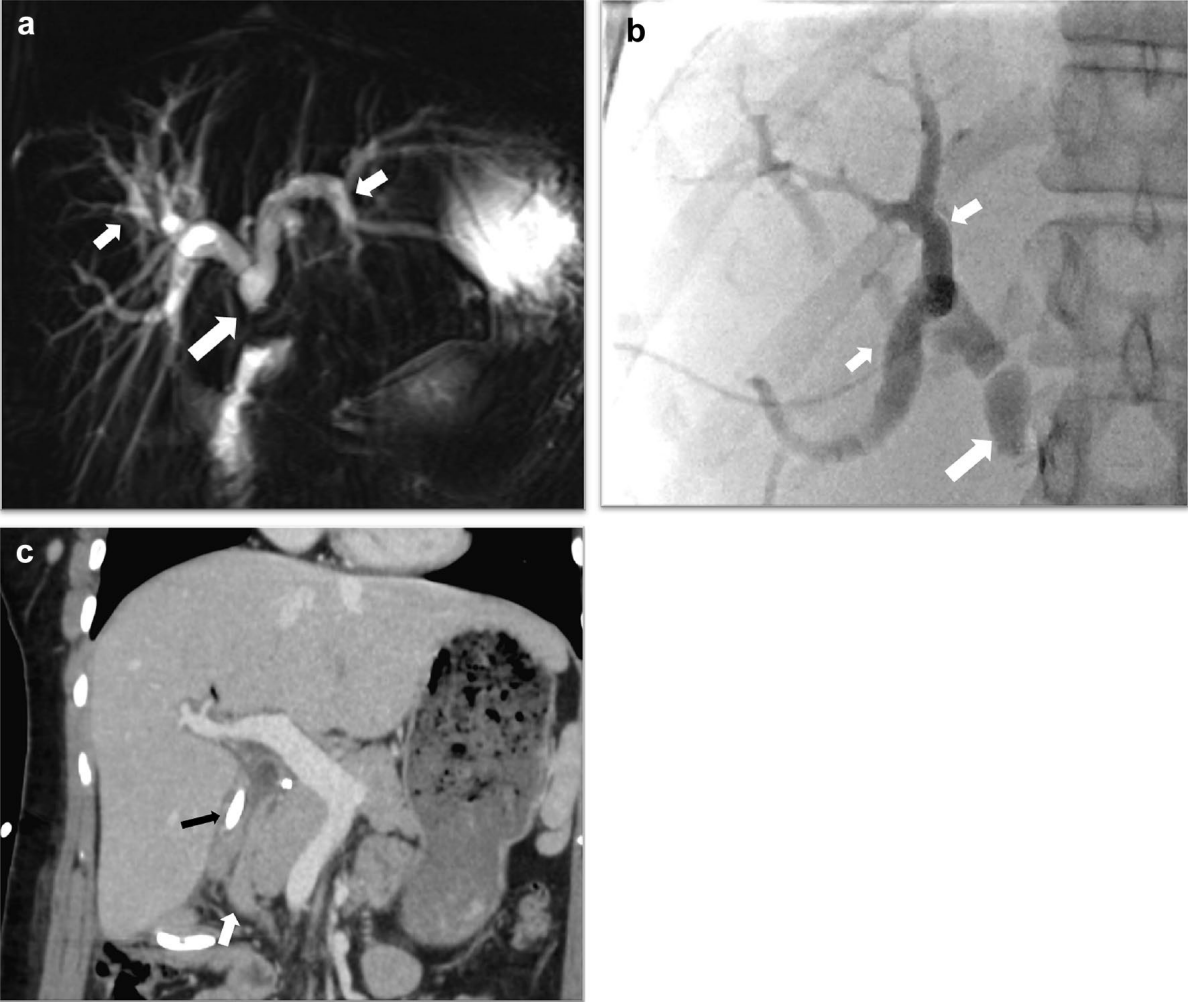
A 28-year-old female with transection of the CBD during cholecystectomy. She presented with jaundice 6 days post-cholecystectomy. a Coronal MRCP and b Percutaneous transhepatic cholangiography in the coronal plane demonstrate abrupt cut-off of the CBD (long white arrow) and biliary dilatation (short white arrows). c) Coronal CT image post hepaticojejunostomy with a Roux-en-Y jejunal loop, which shows a stent within it (black arrow) to the right of the duodenum (white arrow)
